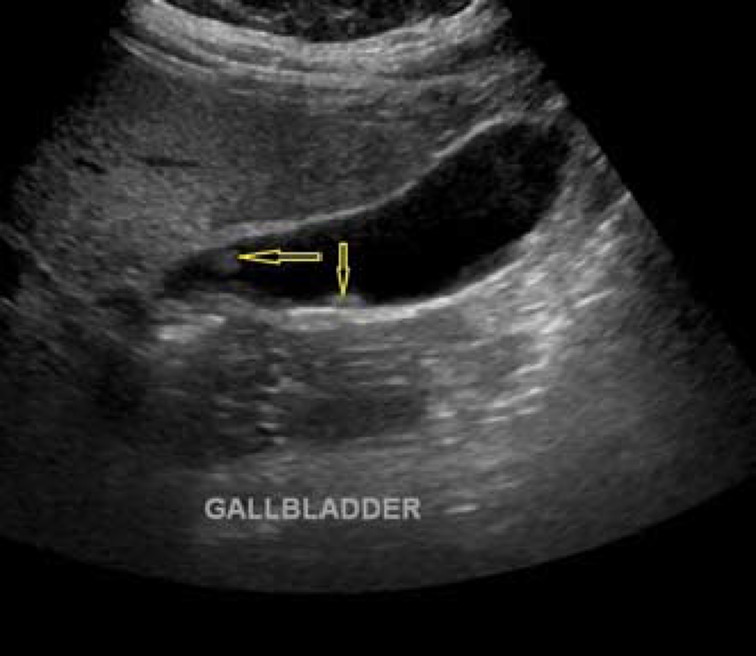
Ultrasound image showing multiple gallbladder polyps (yellow arrows)
